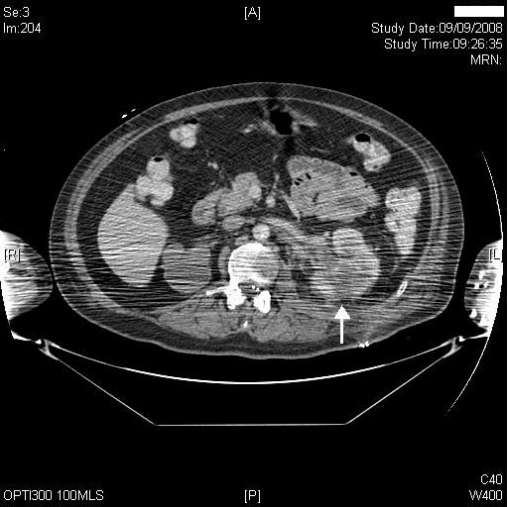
CT of kidneys, performed on 09 September 2008 (arterial phase) showed an area of non-enhancement in posterior cortex (middle third) of left kidney (arrow).
